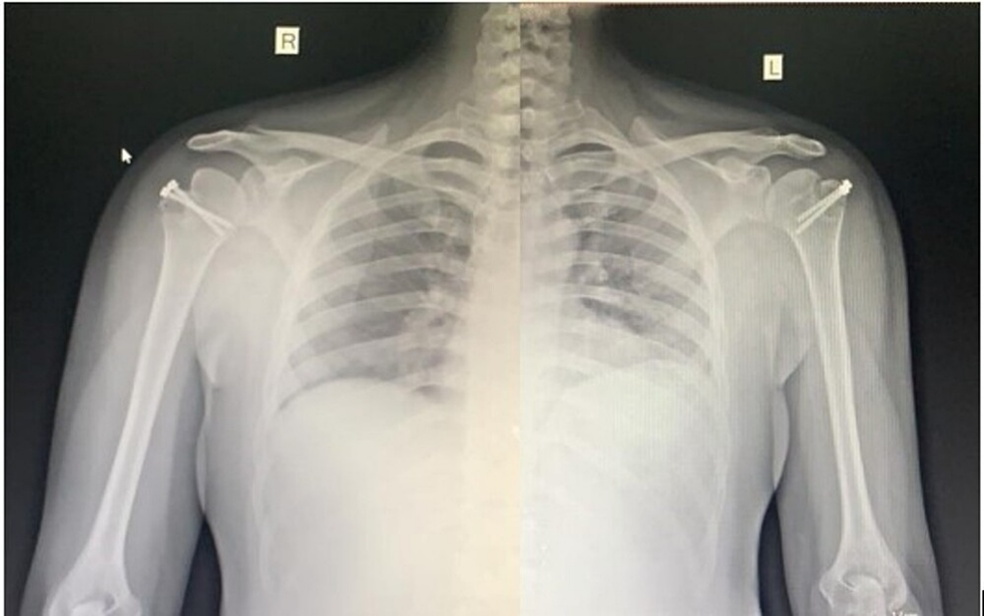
Three months post-operative radiograph
